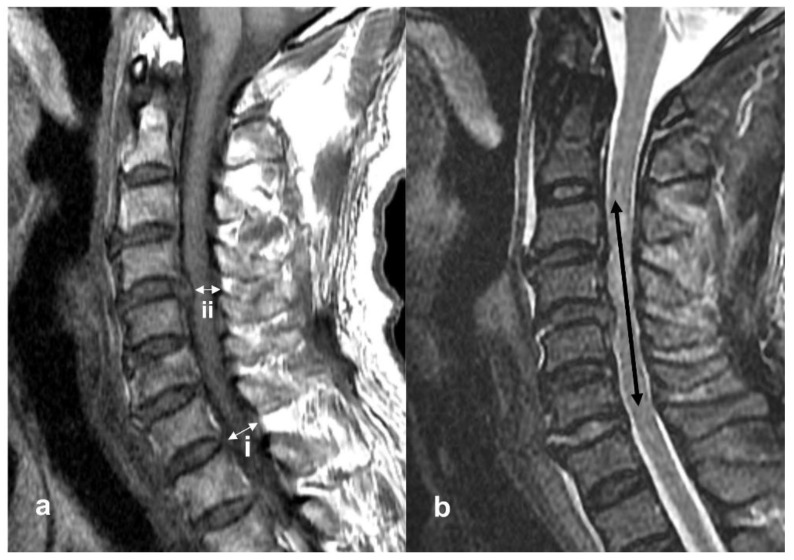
Sagittal magnetic resonance imagery (MRI) obtained in a 69-year-old man who presented with severe adult spinal cord injury without major bone injury and American Spinal Injury Association Impairment Scale (AIS) grade A. (a) T1-weighted image. (i) The diameter of the cervical cord at the non-compression level. (ii) The diameter of the cervical cord at the injured level. Compression rate was calculated by the equation. Compression rate was 35.2%. (b) T2-weighted image at C3-4-5-6 showed the signal change, and the rostrocaudal length of damage was measured in millimeters. Intramedullary lesion length (IMLL) was 62 mm.
